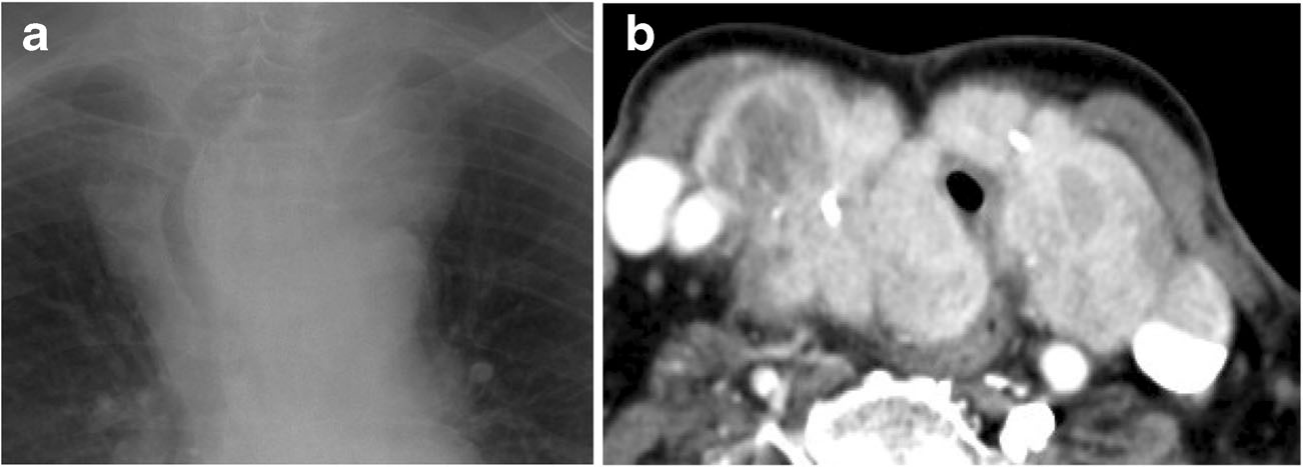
a Radiograph demonstrates tracheal deviation and narrowing at the level of the thoracic inlet. b Axial CT image demonstrates a heterogenous, high attenuating mass with calcifications, consistent with a goiter
